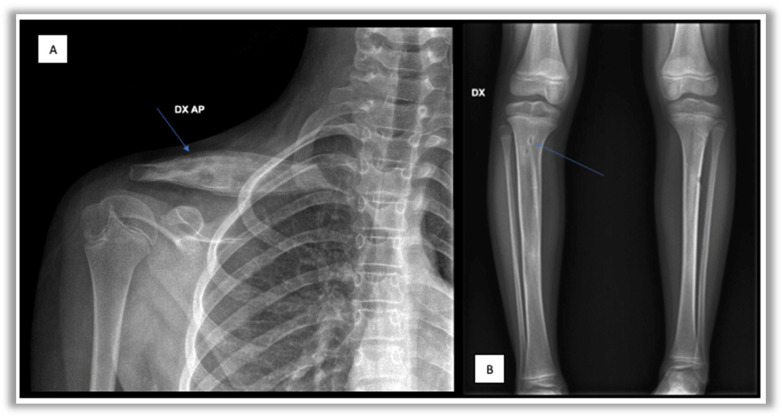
Radiographic images. (A) Right clavicular lesion;(B) right tibial osteolytic lesion.
